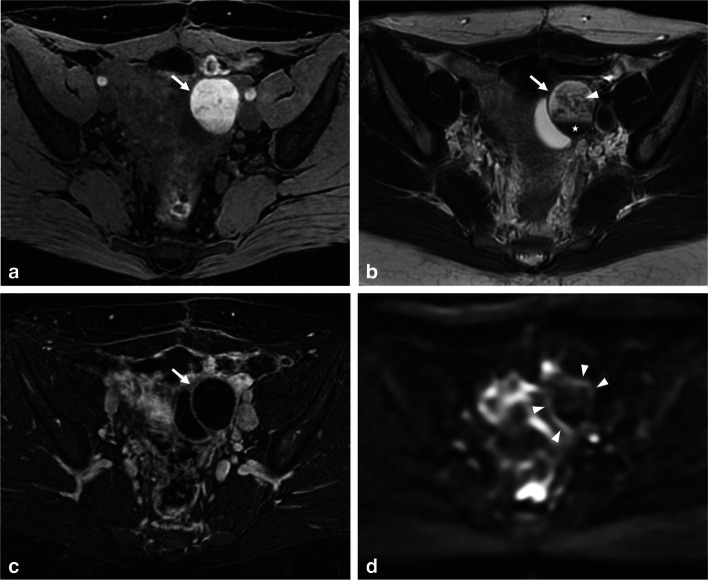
Functional hemorrhagic ovarian cyst in a 28-year-old woman with dysmenorrhea and acute pelvic pain at the time of MRI, and no medical history. a Axial T1-W fat-suppressed MR image shows a T1-hyperintense left hemorrhagic ovarian cyst (arrow). b Axial T2-W MR image shows a heterogeneous cyst (arrow) with upper hypointense lace-like reticular areas (arrowhead) and lower fluid-fluid level (star) with T2 shading. c Axial T1-W contrast-enhanced subtracted MR image shows homogeneous hyperintense rim wall enhancement (arrow). d Axial diffusion-weighted MR image shows an equivalent hyperintense “DWI-ring sign” (arrowheads) compared to c
